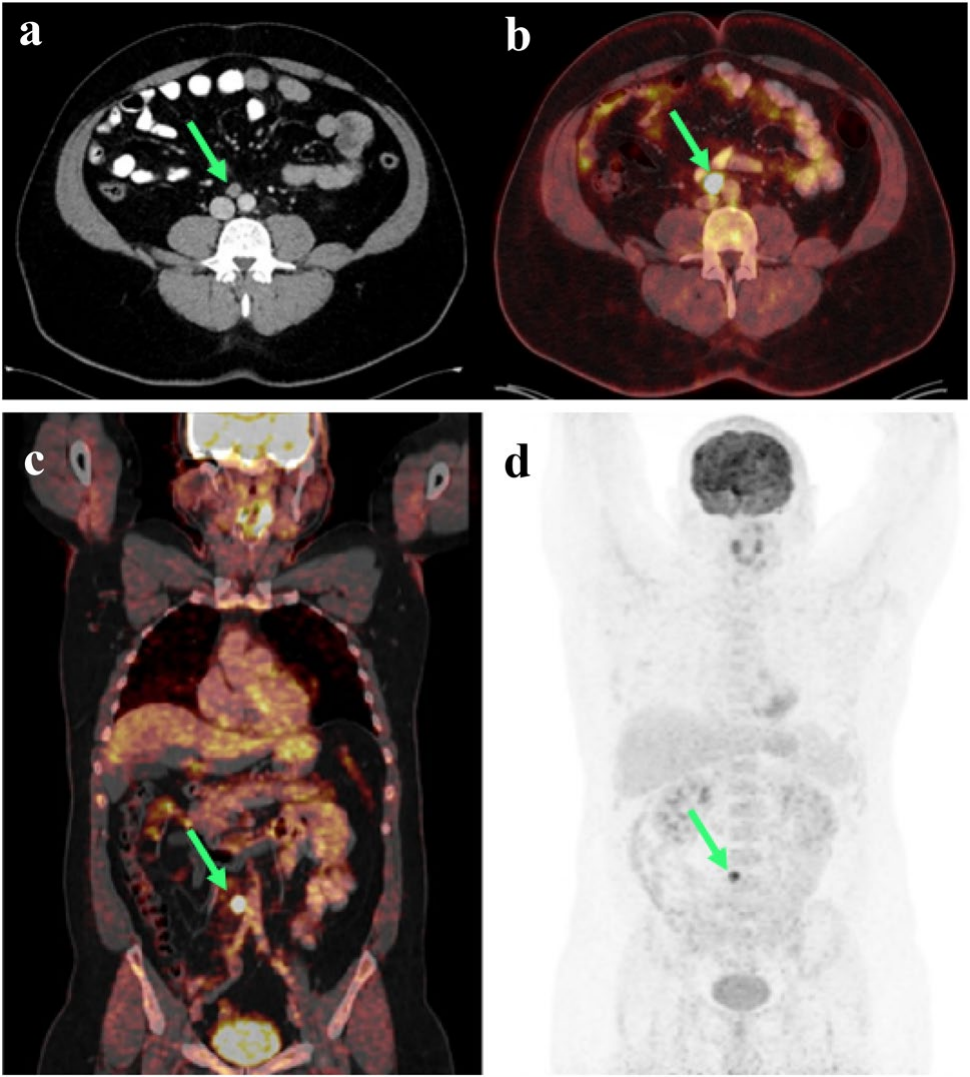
Abdominal and thoracic FDG-PET-CT of an ambiguous retroperitoneal lymph node. a: abdominal CT-Scan. Figure b–d: FGD-PET-CT scan of abdomen/thorax/neck and head with an enlarged interaortocaval lymph node (green arrow) and intense FDG uptake in a patient with right sided seminoma and slightly increased lymph node after orchiectomy (Pictures from “Testicular cancer: Diagnosis and Initial Management”, Springer-Verlag)
